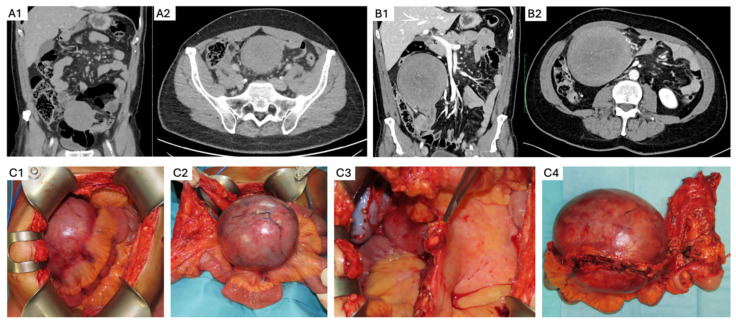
Surgical management of intraabdominal sporadic desmoid tumor. (A1,A2) A 59-year-old male patient presented with a palpable abdominal mass. Biopsy showed a DT, β-catenin-mutated. He underwent active surveillance. (B1,B2) A total of 2 months later, he presented with duodenal obstruction. CT showed rapid progression of the mesenteric DT. (C1–C4) He underwent an uncomplicated mesenteric and small bowel-sparing resection (C1,C2) with minimal margins (C3,C4) and radiologic surveillance, with no recurrence.
